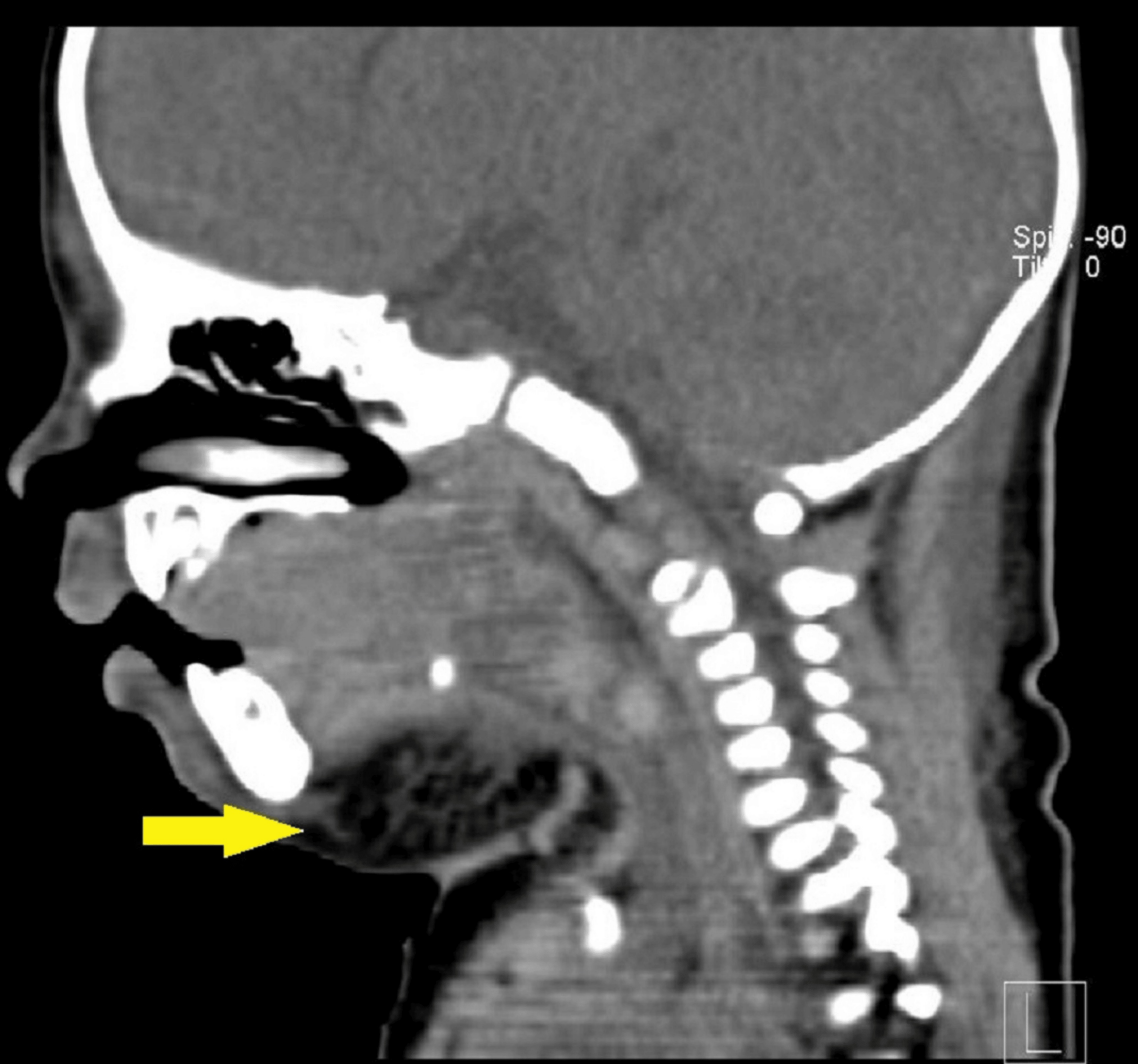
CT neck (sagittal view). Yellow arrow showing submandibular and submental cellulitis.
